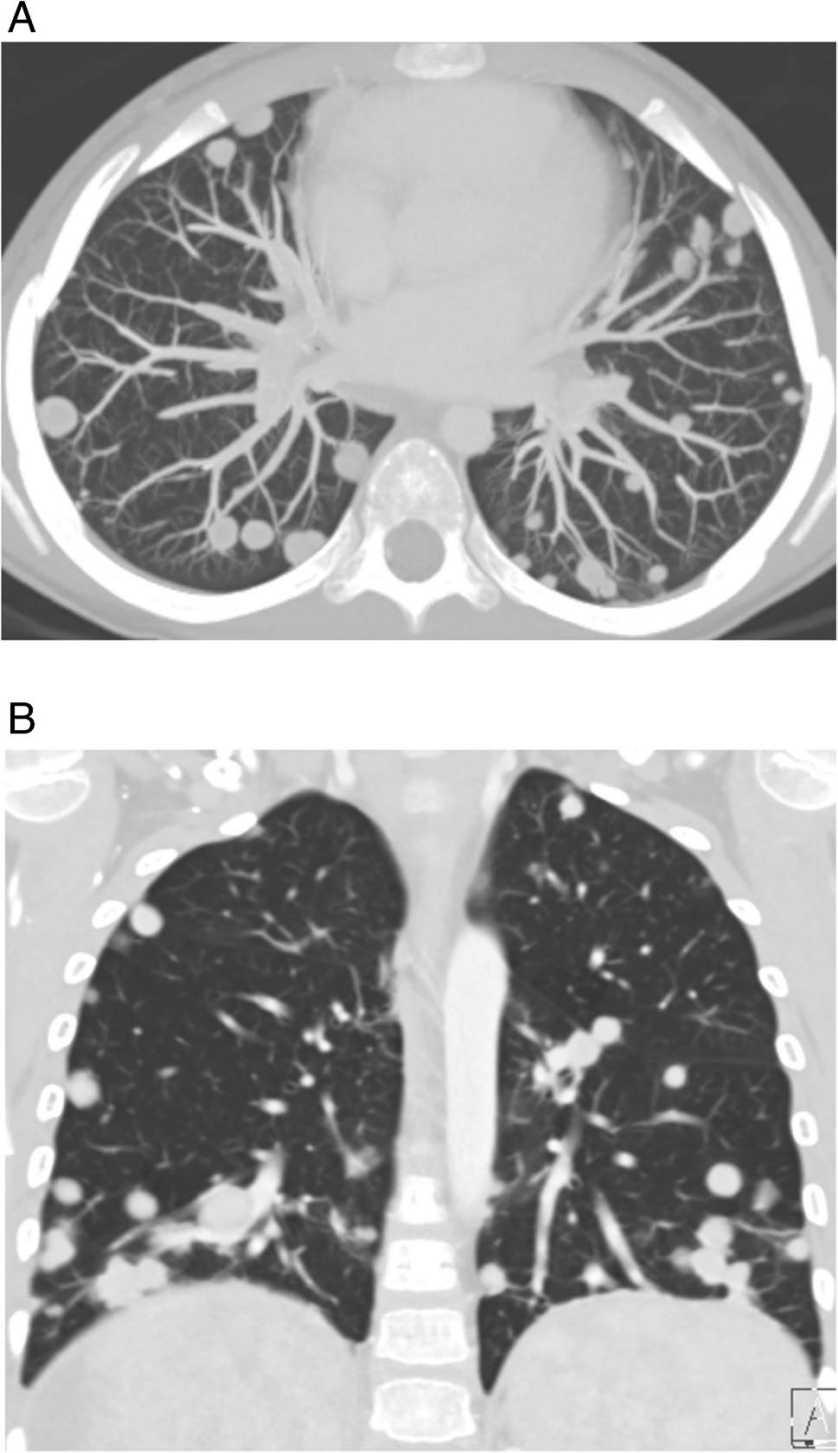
Metastatic hepatoblastoma in a 6-year-old male. Contrast enhanced (A) axial MIP and (B) coronal CT images show numerous, sharply marginated bilateral pulmonary nodules with a peripheral and lower lobe predominant distribution consistent with metastatic disease, confirmed with surgical biopsy
